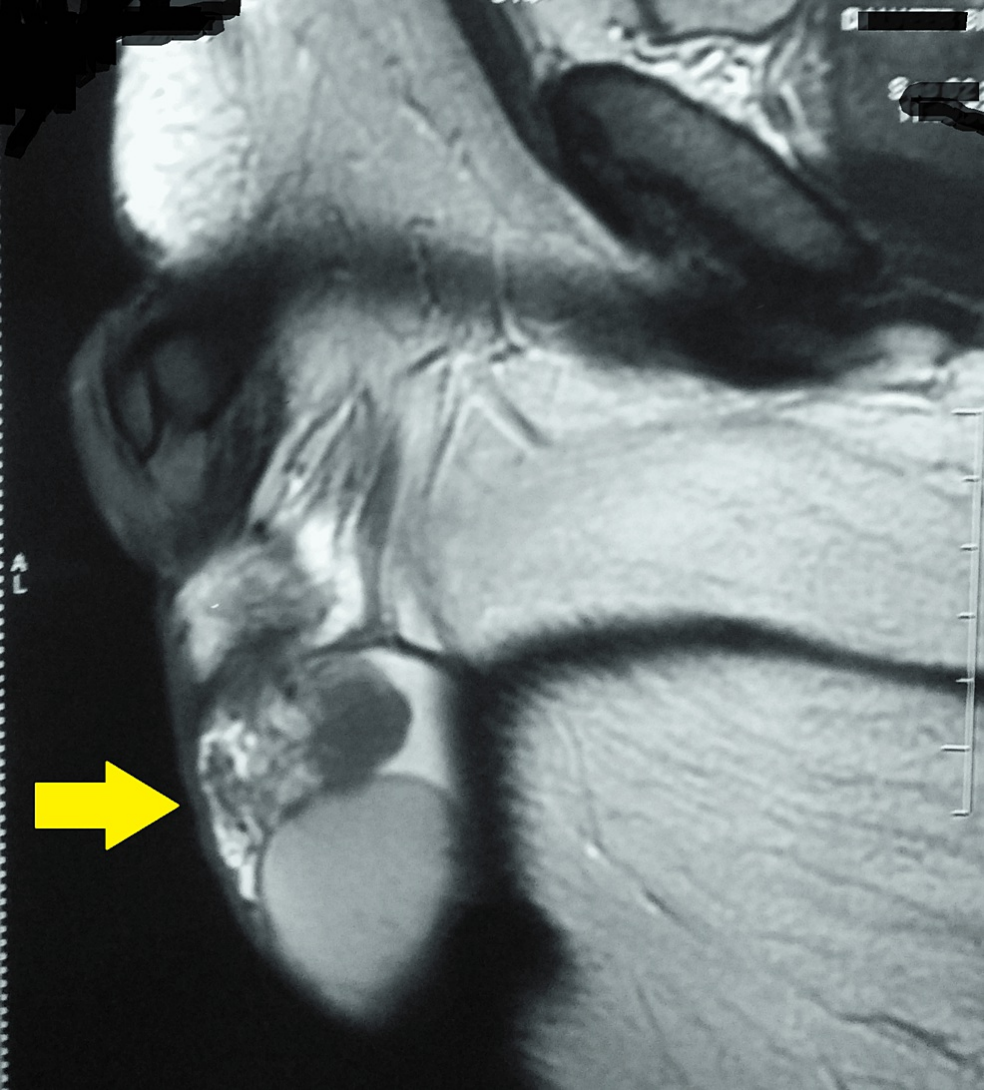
MRI of the scrotum suggestive of nodular thickening in the region of the head of the left epididymis MRI: Magnetic Resonance Imaging
